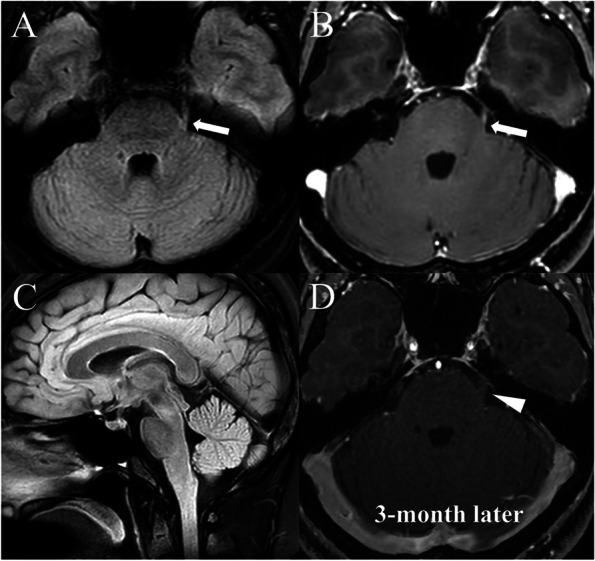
The imaging findings of trigeminal neuritis initial and after treatment. Fluid-Attenuated Inversion Recovery (FLAIR) imaging and gadolinium enhancement T1-weighted imaging shows a high signal intensity lesion in the left trigeminal nerve (arrow) suggestive of trigeminal neuritis (Fig. 1A and 1B). On sagittal FLAIR imaging, there was no evidence of other central nervous system demyelinating disease (Fig. 1C). The follow-up MRI, conducted 3 months later, shows an improved state of the previously observed trigeminal neuritis (arrowhead, Fig. 1D)
